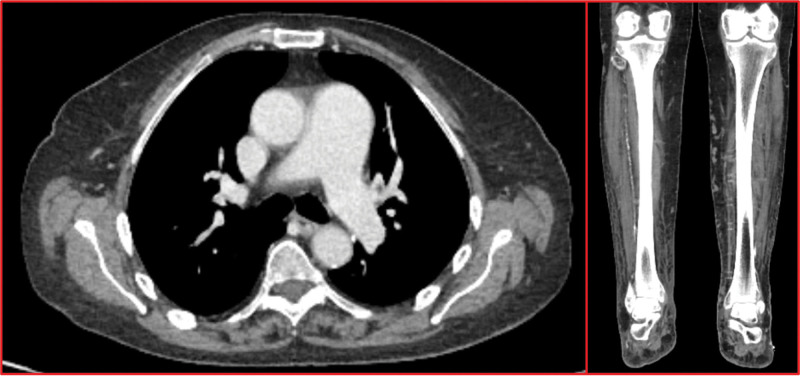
Contrast enhanced CT scan shows main pulmonary trunk enlargement, suggestive for pulmonary hypertension, and right anterior tibial artery narrowing with a beading appearance. CT = computed tomography.
